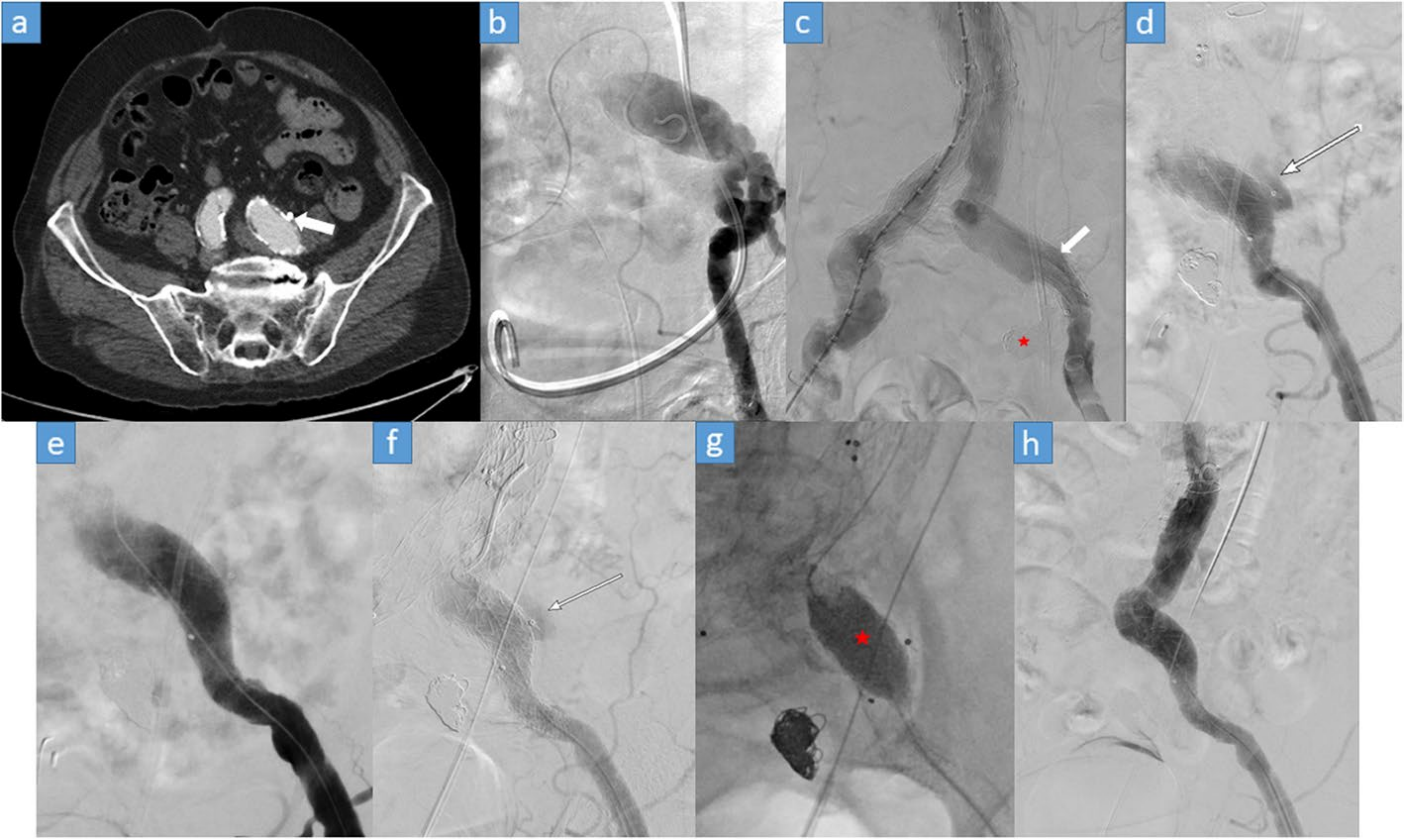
a CT scan showing the point of vascular crossing over the distal CIA with a ureteral stent seen (arrow). b A negative DSA shortly prior to a planned endovascular aortic repair (EVAR) due to an aortic aneurysm. During this angiography session, a kidney bleeding was also excluded (not seen). c Coiling of the IIA (star) and coverage of the origin of the IIA using the left leg of the SG (arrow) were performed during the EVAR procedure. d Pseudoaneurysm at the distal end of the stent graft denoting the first recurrence after 3 years (arrow). e Distal elongation of the SG using a Viabahn VBX SG with complete closure of the pseudoaneurysm in the immediate control image. f DSA after 24 h due to recurrent macrohematuria showing recurrence of the pseudoaneurysm (arrow). g Post-dilatation of the Viabahn VBX SG using a larger balloon (star) at the site of the visible pseudoaneurysm. h Complete obliteration of the pseudoaneurysm
